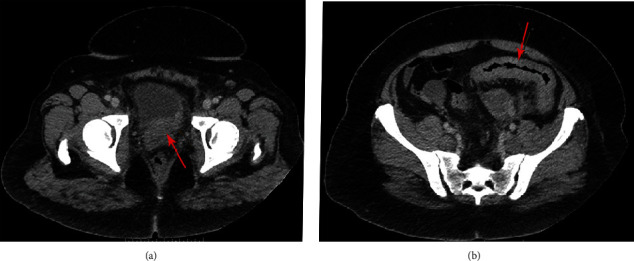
Axial abdominal computed tomography (CT): urinary bladder with thickening of the left posterior wall (a); midportion of sigmoid colon with wall thickening and pericolic infiltration suggestive of acute-subacute segmental colitis (b).
